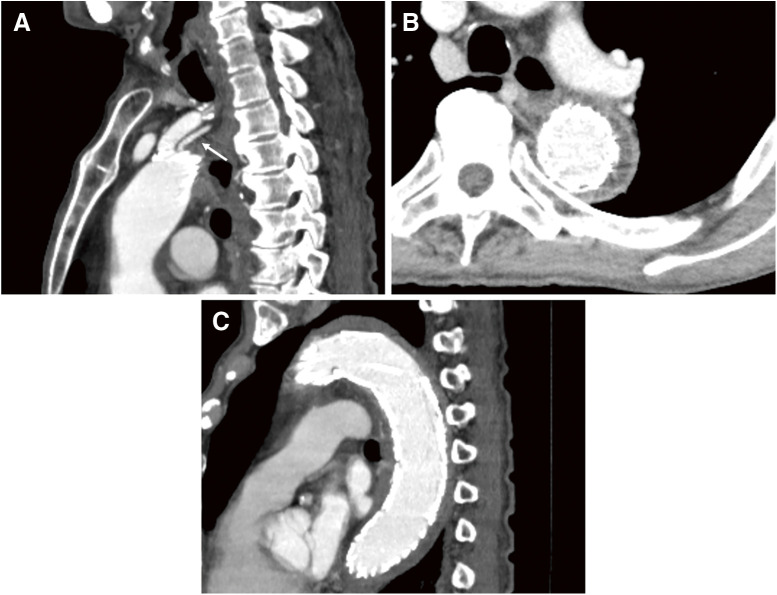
Fig. 3 Postoperative computed tomography. A postoperative computed tomography shows preserved isolated left vertebral artery blood flow (arrow, A); good aortic remodeling and descending aorta diameter reduction to 40 mm (B); and the absence of an ulcer-like projection in the descending aorta (C).
